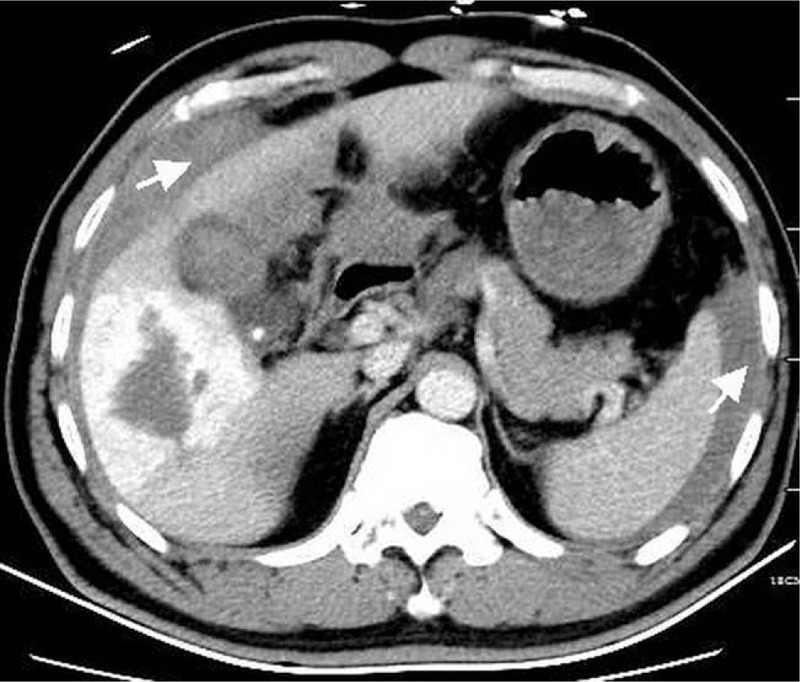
CT with intravenous contrast demonstrates a hepatic hemangioma and free high attenuation fluid around the liver and spleen (*arrow*). CT = computed tomography.
